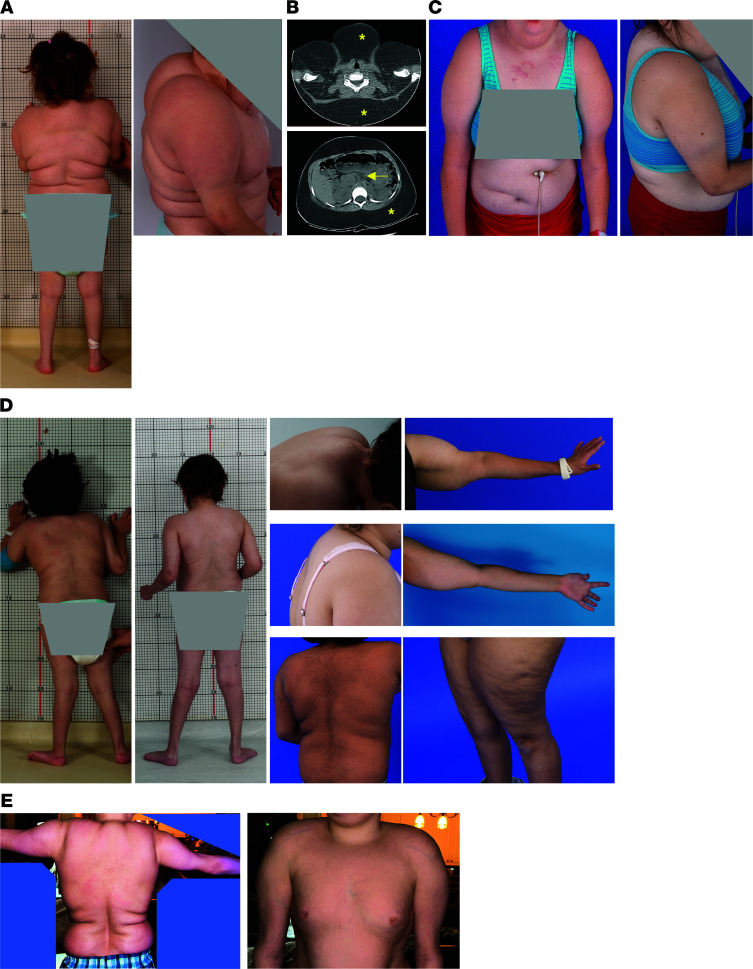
Subcutaneous fat accumulation with lipodystrophy of lower extremities in MMA. (A) Cushingoid phenotype of the index patient, a 6-year-old White girl, with fat accumulation in the torso, dorsocervical area, and shoulders/upper arms, with lipodystrophy of the distal arms and legs. (B) Axial sections of cervical and abdominal CT imaging demonstrating accumulation of subcutaneous fat, marked with asterisks. Arrow points to the minimal visceral fat present in the abdominal CT section. (C) Index patient at 18 years of age showing subcutaneous fat depots over her torso and upper arms. (D) A similar fat accumulation in proximal upper and lower limbs with wasting in distal extremities and presence of dorsocervical hump in 7 additional male and female patients with *mut^0^* (low to no methylmalonyl-CoA enzymatic activity) or *MMUT*-type and (E) 1 *cblB*- or *MMAB*-type MMA of various racial and ethnic backgrounds (White, Asian, Pacific Islander, and Hispanic).
